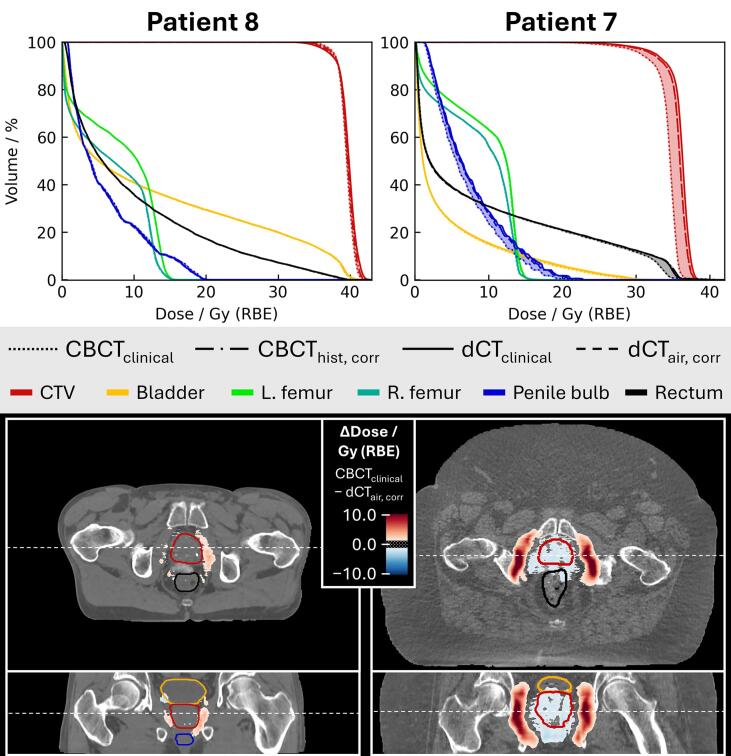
Differences in proton dose calculation for two patients with different BMI. Top: Fraction doses (scaled by a factor of 5) compared in terms of DVHs for each CBCT-based image of a single fraction. Bottom: Corresponding dose differences (CBCT_clinical_ minus dCT_air, corr_) superimposed on the axial and coronal views of CBCT_clinical_. Differences between −1 and 1 Gy (RBE) are not visualized. The dashed lines indicate the locations of the respective axial and coronal slices. Overshooting of the dose calculated on CBCT_clinical_ is evident, resulting in a considerably lower predicted target coverage for patient 7 compared to dCT_air, corr_.
